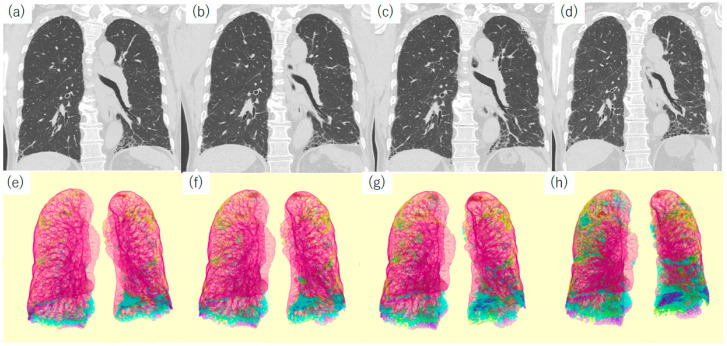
CT images and their segmented images of a 70-year-old male patient with IPF (UIP pattern was proven by surgical lung biopsy.) (a,e) Initial CT, (b,f) CT taken two years later, (c,g) CT obtained four years later, and (d,h) CT after six years. All CTs were acquired at a standard dose. Segmentation was performed using QZIP-ILD (Ziosoft Inc.). Segmentation color codes: pink, normal lung tissue; green, GGO, blue, reticulation; yellow, consolidation with traction bronchiectasis; and violet, honeycomb pattern. In the initial CT image, a small amount of honeycomb pattern can be observed bilaterally at the lung base. Abbreviations: CT, computed tomography; GGO, ground-glass opacity; IPF, idiopathic pulmonary fibrosis; and UIP, usual interstitial pneumonia.
